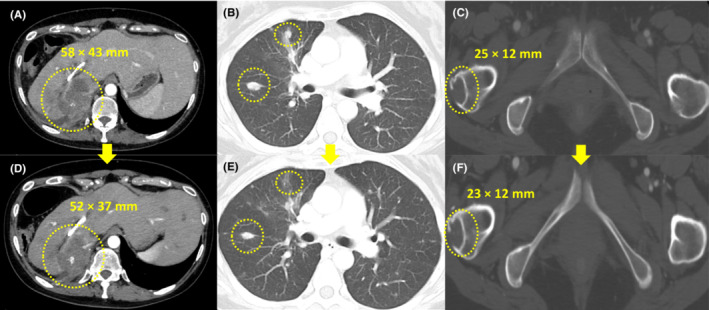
Two months after initiating pemigatinib. Computed tomography scan showing the primary tumor in the intrahepatic region, lung, and bone metastases before pemigatinib treatment (A–C). Two months after initiating pemigatinib (C–E), all lesions were determined to be stable disease (RECIST version1.1) with a reduced length diameter within 20%. RECIST, Response Evaluation Criteria in Solid Tumors.
